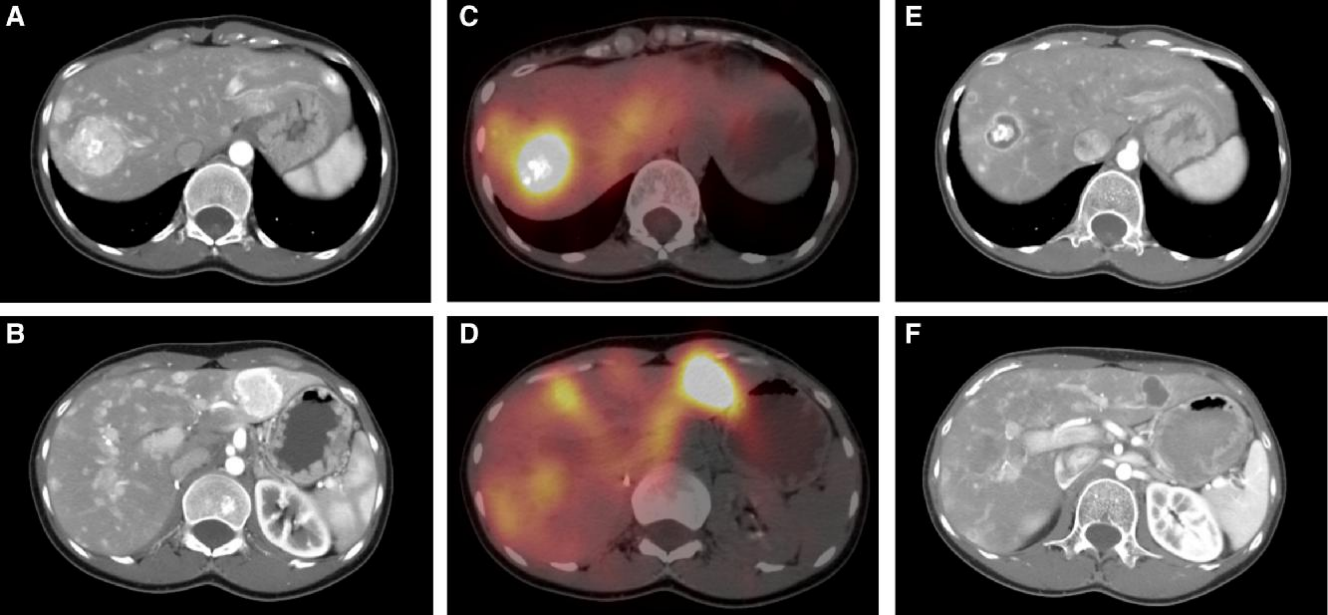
Liver metastases before (A and B), immediately after (C and D) and 5 months after (E and F) transarterial radioembolization with Y90-SIR-spheres. SPECT/CT images (C and D) show significant radiation emission from dominant liver metastases immediately following therapy.
